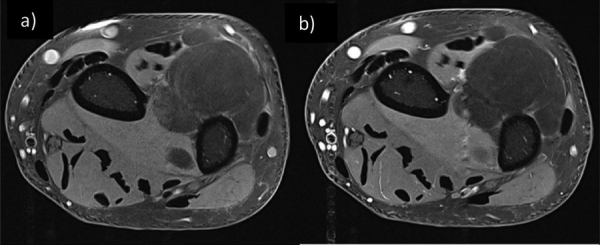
Axial T1-weighted image with fat saturation before (a) and after intravenous gadolinium injection (b).
